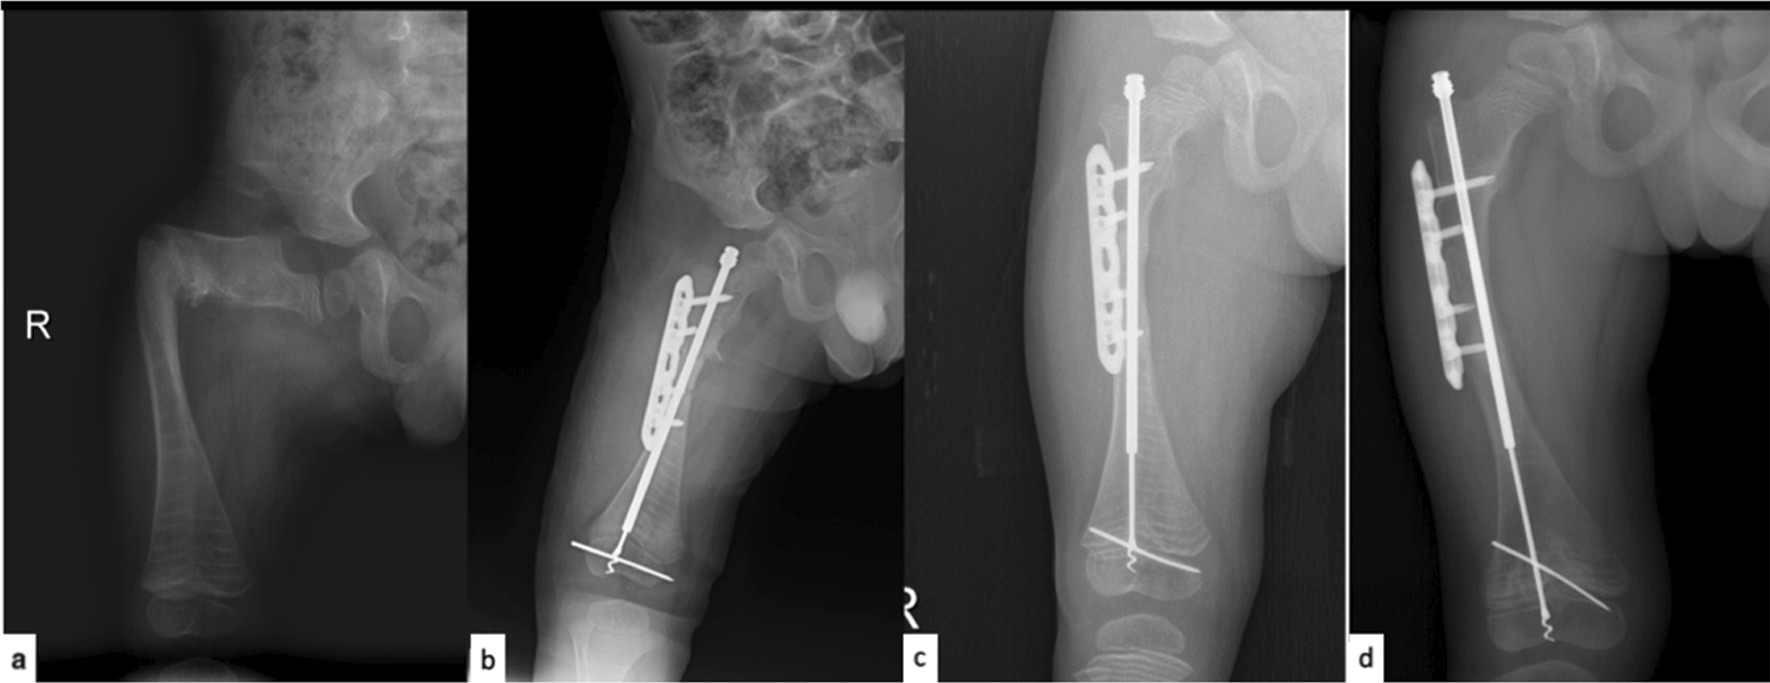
Patient with intramedullary fixation combined with plate and screws a preoperative radiograph, b postoperative first-day radiograph, c second-year follow-up radiograph, d third-year follow-up radiograph
